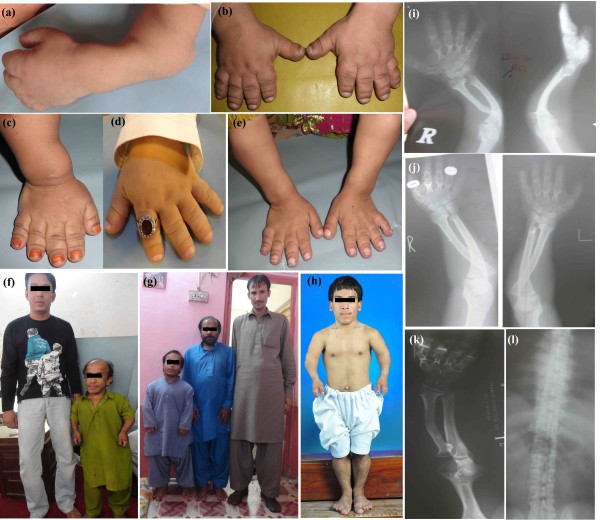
Clinical features of AMDM. Bowing of forearm in an affected member (V-8) in family A (a), affected members including V-5 in family B, V-4 in family C, VI-6 in family D, V-2 in family E showing short fingers and redundant skin (b-e), an affected member V-4 at 38 years of age with his younger brother V-5 at 27 years of age in family A (f), an affected member V-3 at 36 years of age with a carrier VI-1 at 29 years of age in family D along with one of the authors (g), an affected member IV-5 in family F showing short stature and short extremities (h). Radiograph of hand and forearm of an affected member V-4 in family A (i), V-3 in family D (j), IV-5 of family F (k), showing epiphysis of the radius, shortening of ulna, short and stubby metacarpels. Radiograph of vertebral column of an affected member IV-5 in family F showing mild platyspondyly (l).
